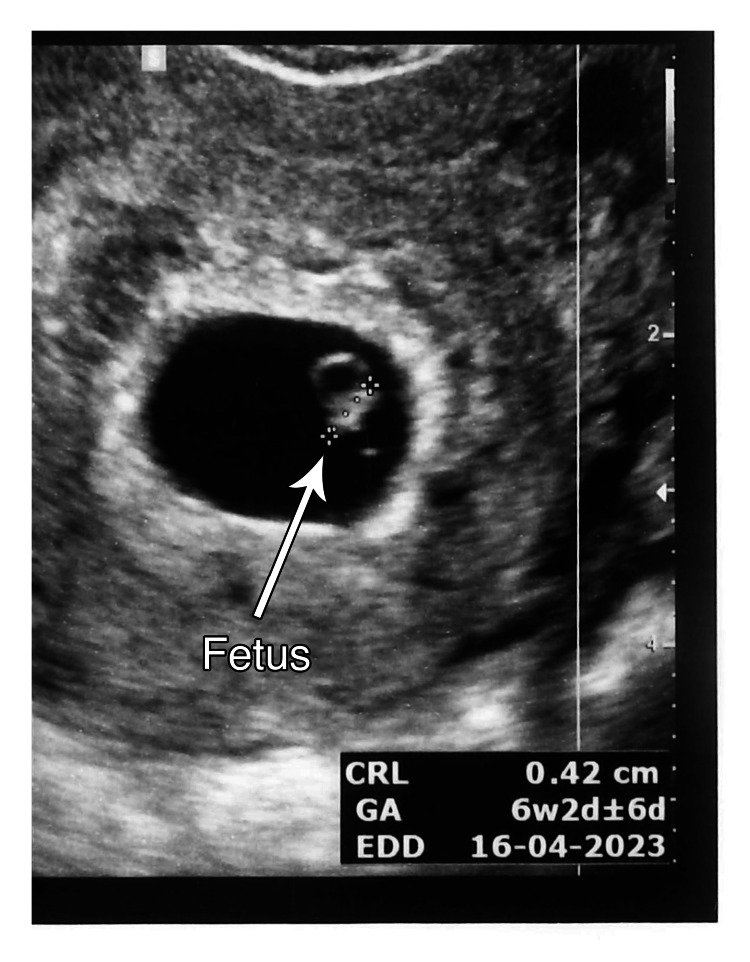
TVUS image of fetus (CRL 0.42 cm = 6 weeks and 2 days) TVUS: transvaginal ultrasound; CRL: crown-rump length
